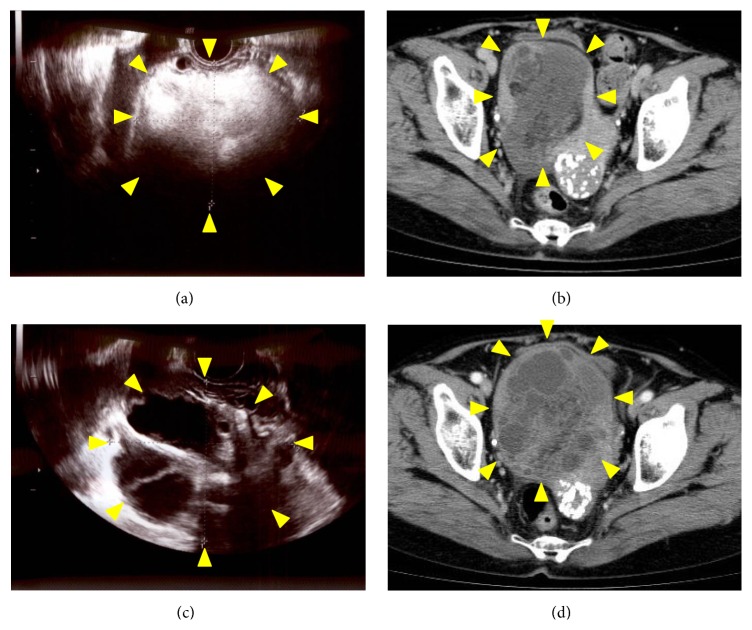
On referral to our institution, transvaginal ultrasonography (a) and computed tomography (CT) (b) demonstrated a 10 cm pelvic tumor. Three months later, transvaginal ultrasonography showed a multilocular tumor (c) that showed heterogeneous enhancement on contrast-enhanced CT (d). Arrowheads indicate the pelvic tumor.
